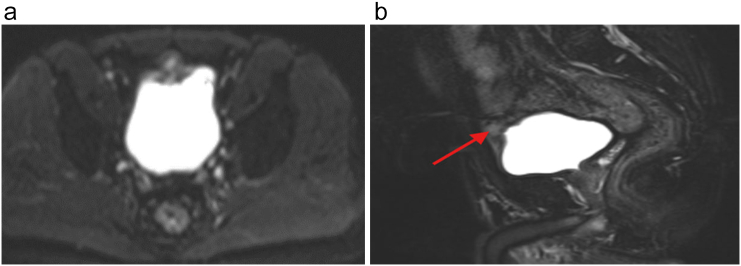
MRI of the patient shows bladder dome mass.
